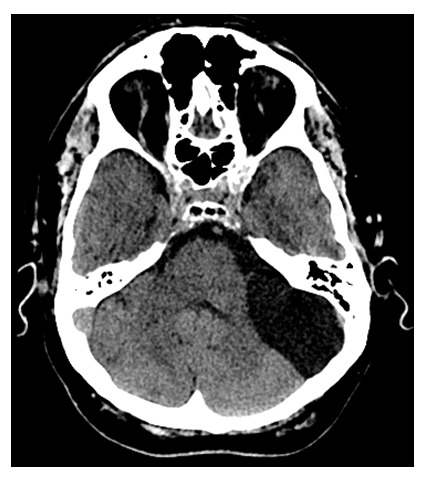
Head computed tomography (CT) scan revealing a large left extra-axial cystic lesion that was being monitored before current symptomatology.
